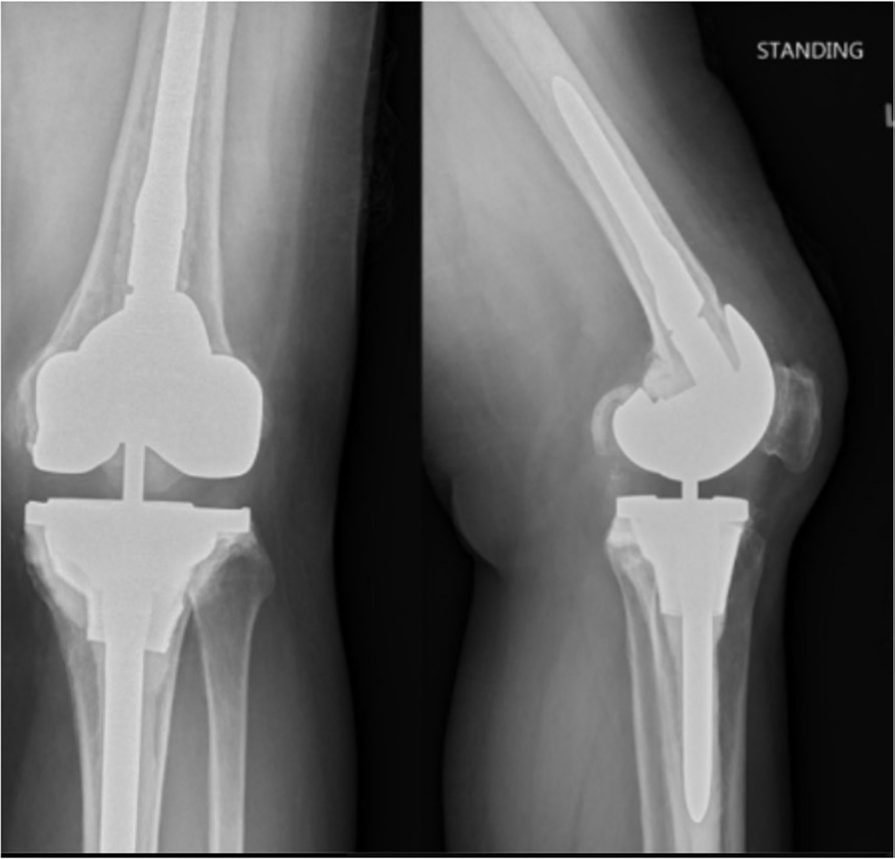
Postoperative radiograph of a case receiving metaphyseal cone using free-hand burring technique
